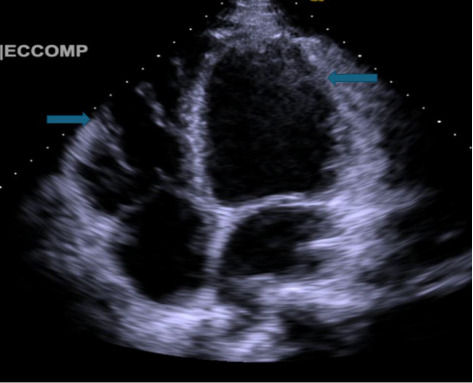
Four-chamber view on echocardiogram showing dilated left ventricle.
